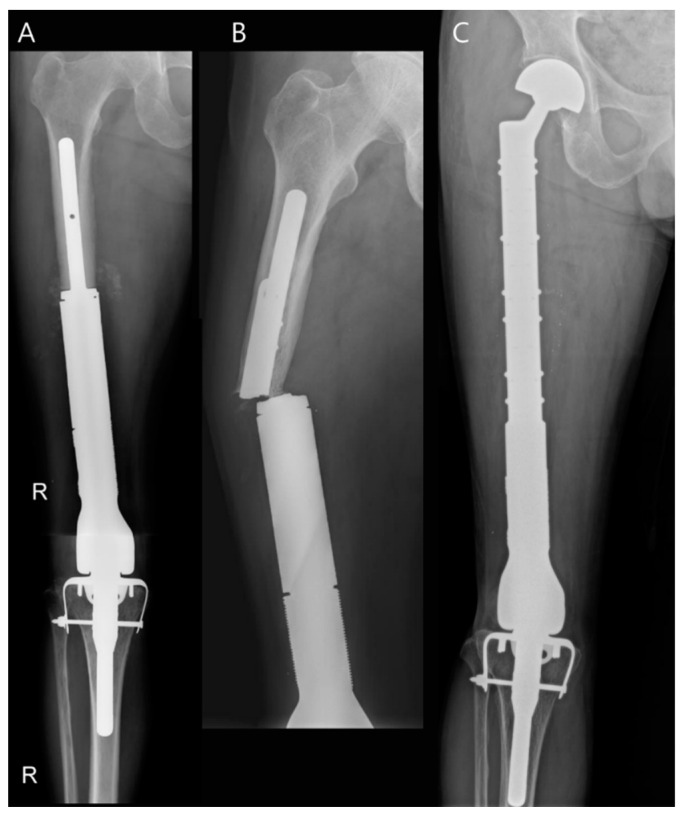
Mechanical failure following revisional limb-salvage surgery for osteosarcoma. (A) Immediate postoperative plain radiograph is shown (The letter R is an abbreviation for right). (B) Follow-up plain radiograph shows a fracture at the base of a prosthetic stem. (C) Plain radiograph after revisional limb-salvage surgery is shown.
